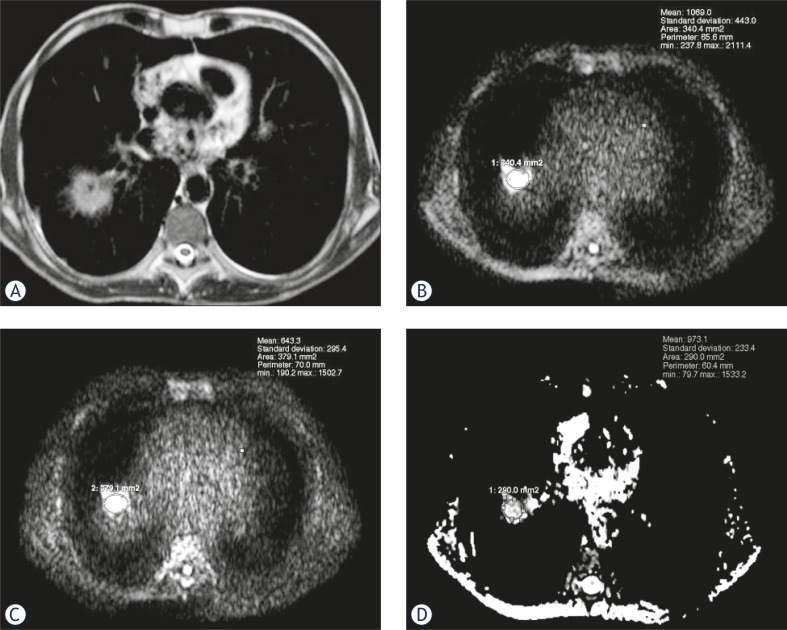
A 56-year-old man with poorly-differentiated non-small cell carcinoma. (A) Axial T2-weighted MR image shows mass in the right lung. (B) diffusion-weighted magnetic resonance image with b value of 500 sec/mm^2^ shows hyperintense mass (SI = 1069). (C) diffusion-weighted magnetic resonance image with b value of 1000 sec/mm^2^ shows hyperintensity of the mass is still remarkable (SI = 643). (D) ADC map shows ADC value is (0.97×10^−3^) sec/mm^2^.
